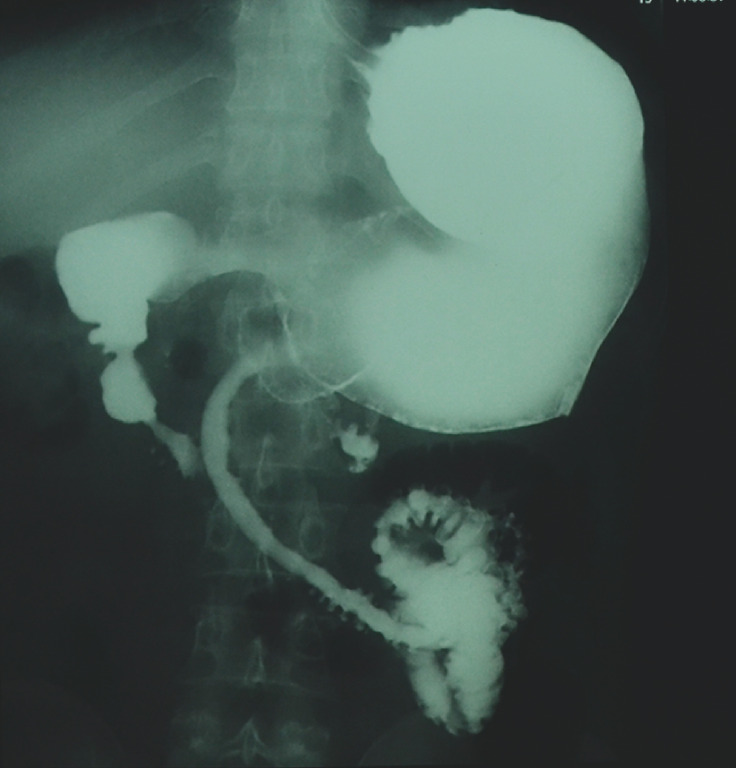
Radiographic image from the barium meal follow-through showing a long-segment stricture (22 cm) in the efferent loop, suggestive of active eosinophilic gastroenteritis.
